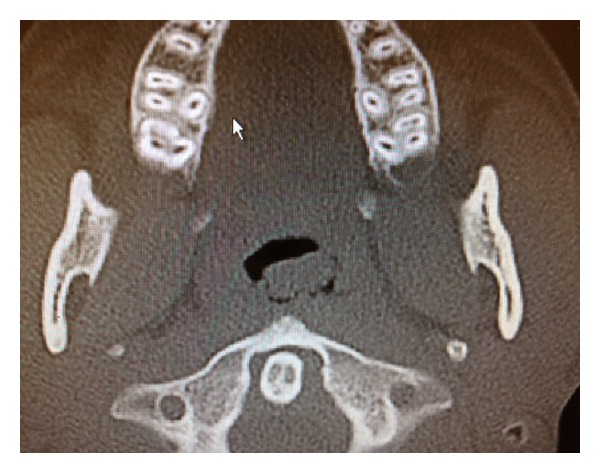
CT axial image demonstrating extension of soft tissue from the nasopharynx on the left side.
